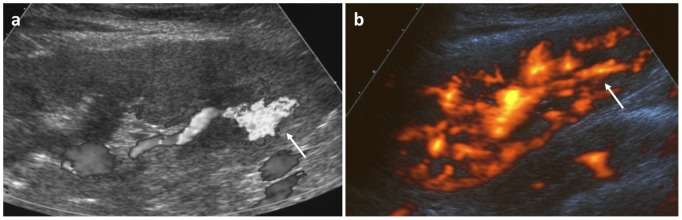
Ultrasound findings of an AV fistula (white arrow). The fistula showed spontaneous regression (13 year old patient, female).
